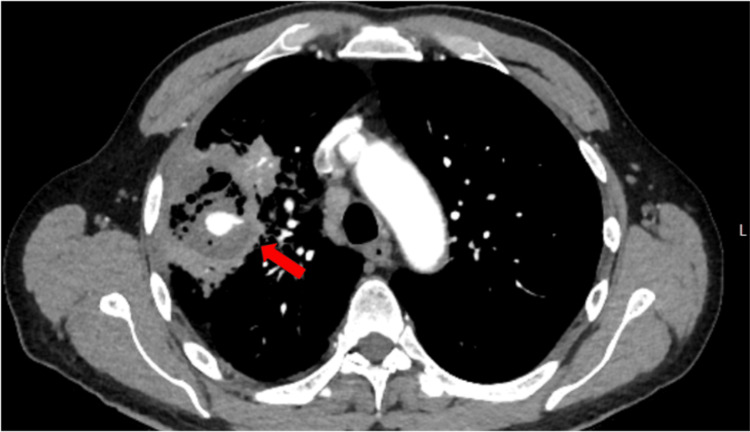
CTA chest axial view demonstrating an irregular right upper lobe complex cavitary lesion with internal contrast extravasation compatible with a pulmonary artery pseudoaneurysm and hemorrhage, consistent with a Rasmussen aneurysm (red arrow).
